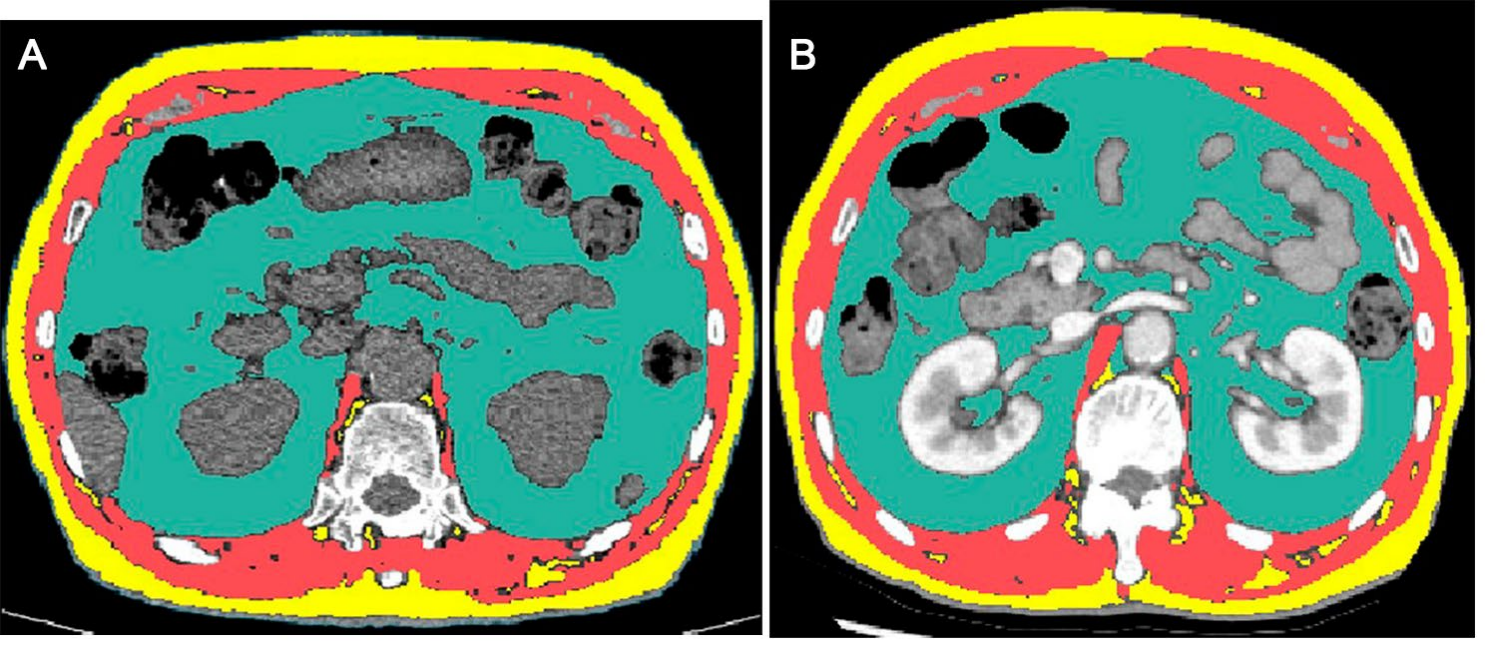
Representative case of fully automated CT-based body composition analysis in (A) baseline and (B) one-year follow-up CT images in a patient belonging to the lowest quartile of the change in fat area. Axial images at the T12-L1 vertebral level demonstrate visceral fat (green), subcutaneous fat (yellow), and skeletal muscle (red). Between CT images, the fat area decreased by 89.9 cm^2^ (baseline: 370.0 cm^2^, one-year follow-up: 280.1 cm^2^). This patient died 10 months later despite receiving a lung transplantation
